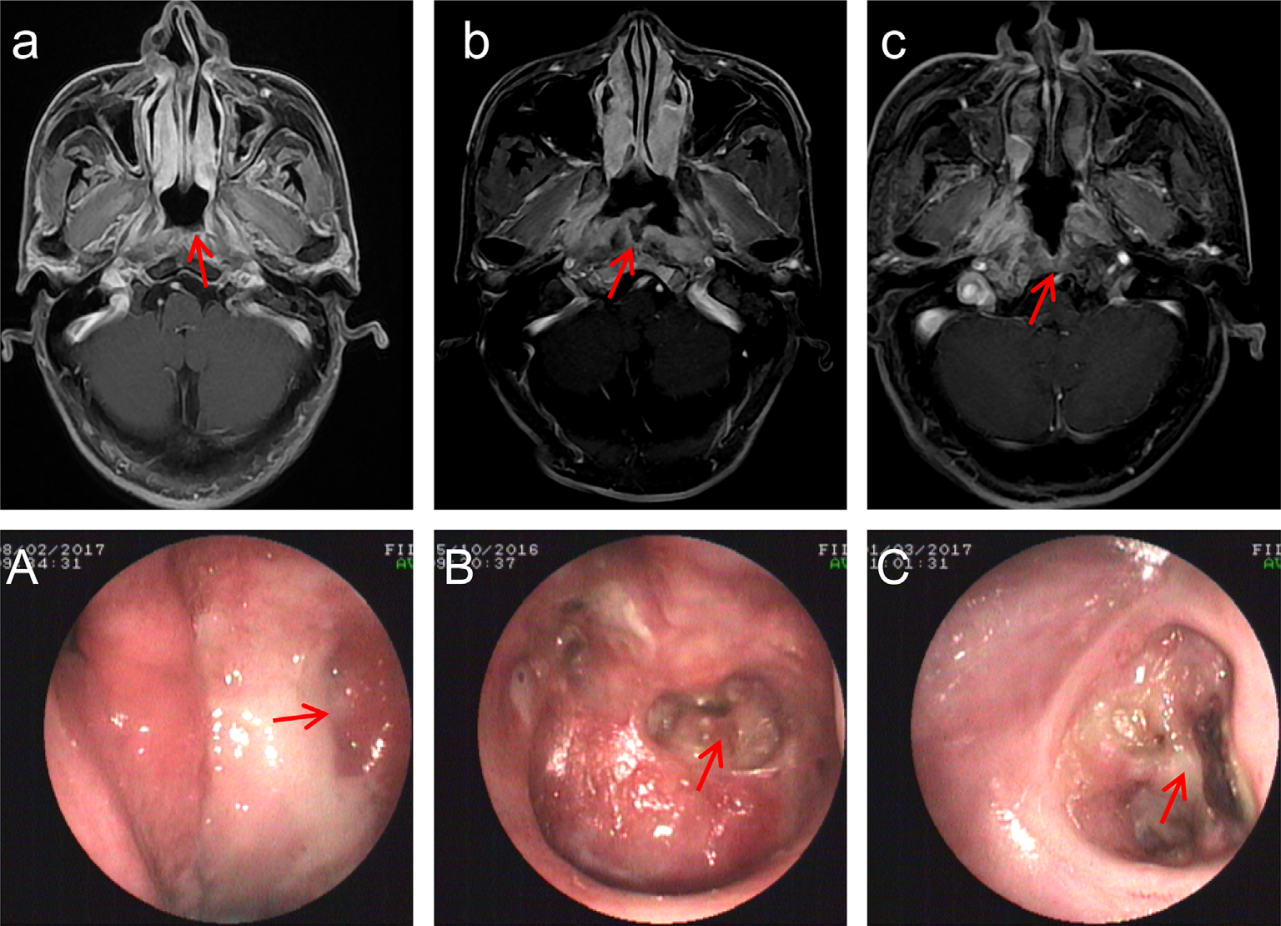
MRI and endoscopic images with post-radiation nasopharyngeal necrosis (PRNN). (a) Transverse, contrast-enhanced T1-weighted magnetic resonance imaging (MRI) of mild-grade PRNN shows discontinuous nasopharyngeal mucosa lines. (A) Endoscopic image with mild-grade PRNN. (b) Transverse, contrast-enhanced T1-weighted MRI of moderate-grade PRNN shows discontinuous mucosa lines and non-enhanced areas. (B) Endoscopic image with moderate-grade PRNN shows a superficial ulcer. (c) Transverse, contrast-enhanced T1-weighted MRI of severe-grade PRNN shows invasion of the skull base bone. (C) Endoscopic images with moderate-grade PRNN show a deep ulcer. The red arrow points to the lesion.
